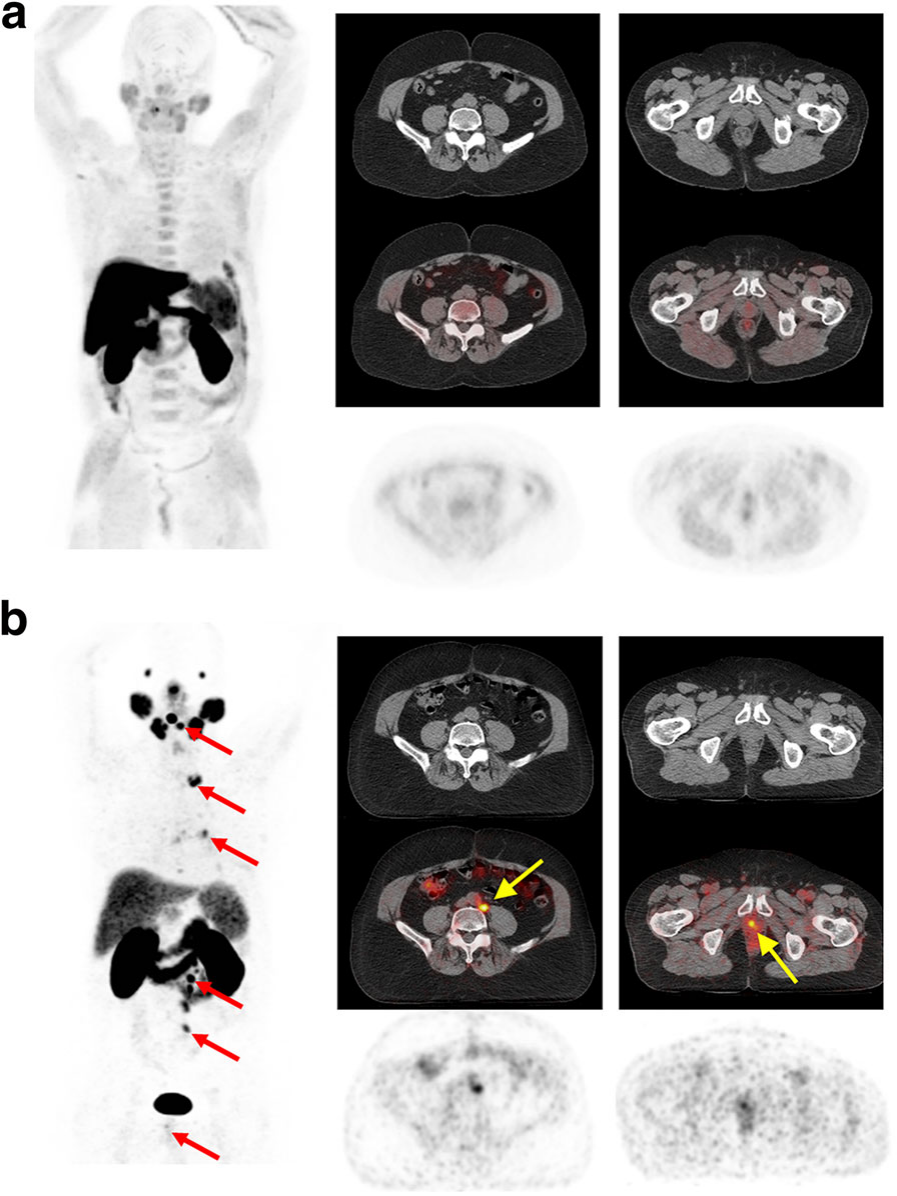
Patient 5. A 68-year-old patient with PCa treated with radiotherapy and complete androgen blockade with current elements of biochemical relapse (PSA level 2.33 ng/ml), scanned with ^11^C-Choline (a) and with ^68^Ga-PSMA (b).Several metastases are demonstrated only by means of ^68^Ga-PSMA alone (red arrows). Yellow arrows show a metastatic retroperitoneal lymph node and a local relapse in ^68^Ga-PSMA PET/CT, while ^11^C-Choline PET/CT showed no suspicious lesion in these areas
